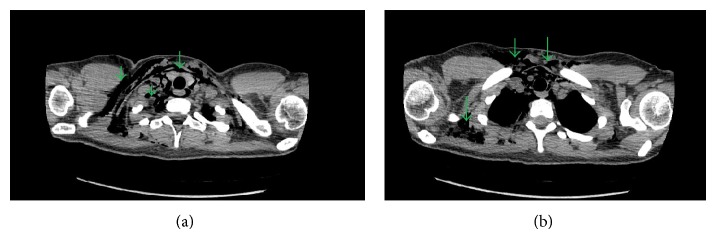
These images are obtained from CT thorax without contrast (mediastinal window) which confirms the findings in the chest X-ray (Green arrows).
